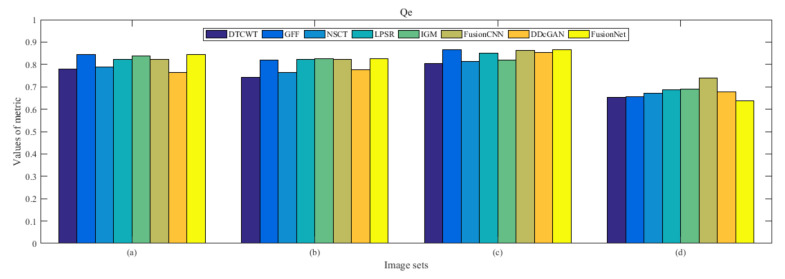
Values of $Q_{e}$ in the fused images (MRI-SPECT): (a) is composed of eight images in Figure 8, (b) is composed of eight images in Figure 9, (c) is composed of eight images in Figure 10, (d) is composed of eight images in Figure 11.
